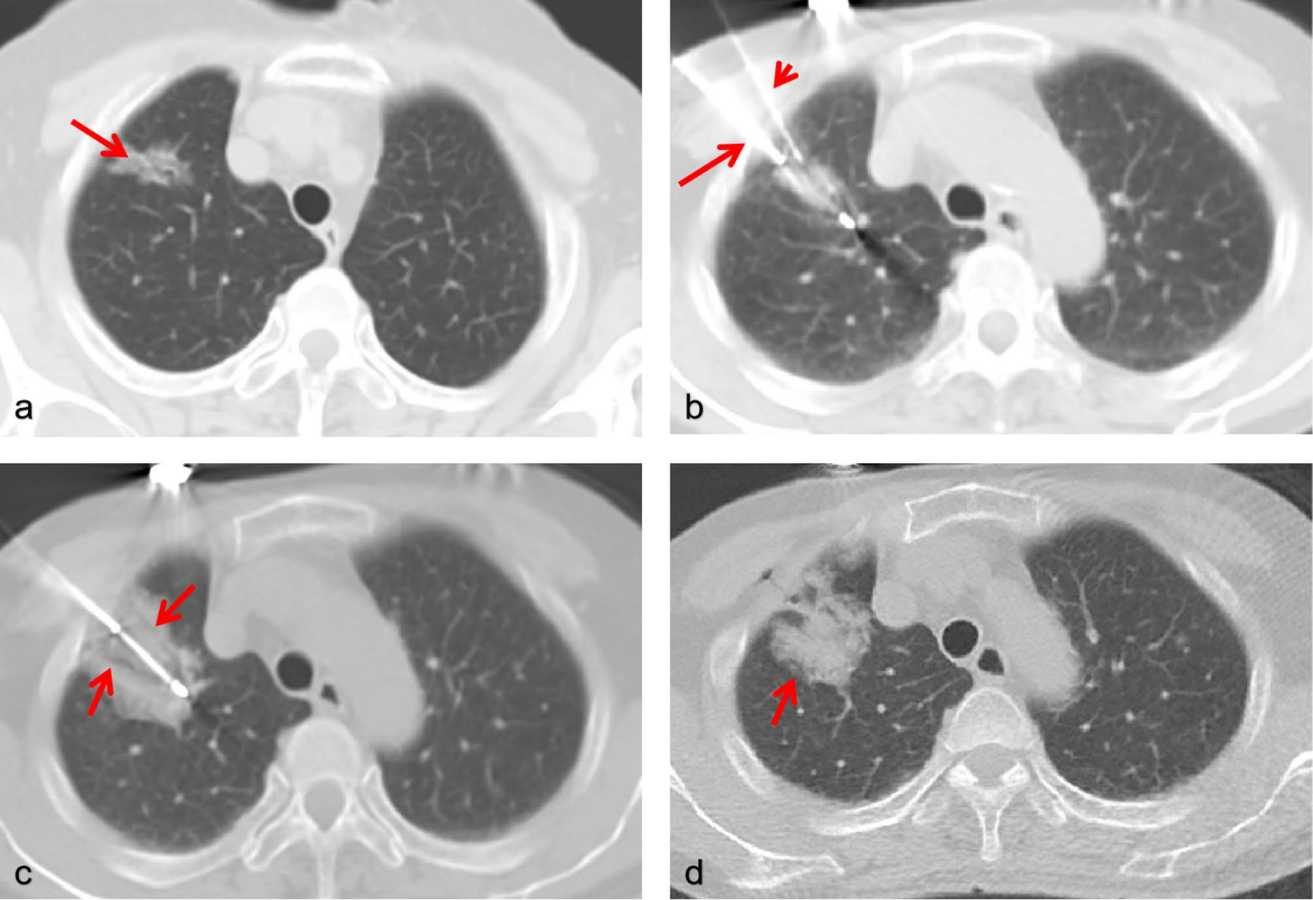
Synchronous procedure in a 71-year old woman. (a) Computed tomography image showing a single sub-solid nodule (arrow), 20 mm ×12 mm, in the apical segment of the right upper lobe, irregular margin, and pleural tail sign. (b) A cryoablation probe (long arrow) was placed into the nodule while the biopsy needle was at the edge of the lesion (short arrow). (c) During the cyroablation procedure, the locus center began to occur liquefactive necrosis as well as the ice ball emerged (arrows). (d) In the thoracic computed tomography scan immediately after cryoablation, the ablated nodule presented with a circular ground-glass opacity (arrow). Finally, the pathological result showed it an early stage lung adenocarcinoma
